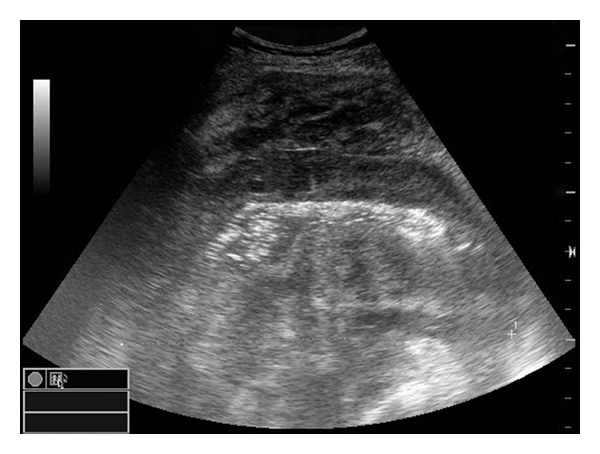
Ultrasonographic image displaying solid areas consistent with thickening of the intestinal wall in the right-lower quadrant.
